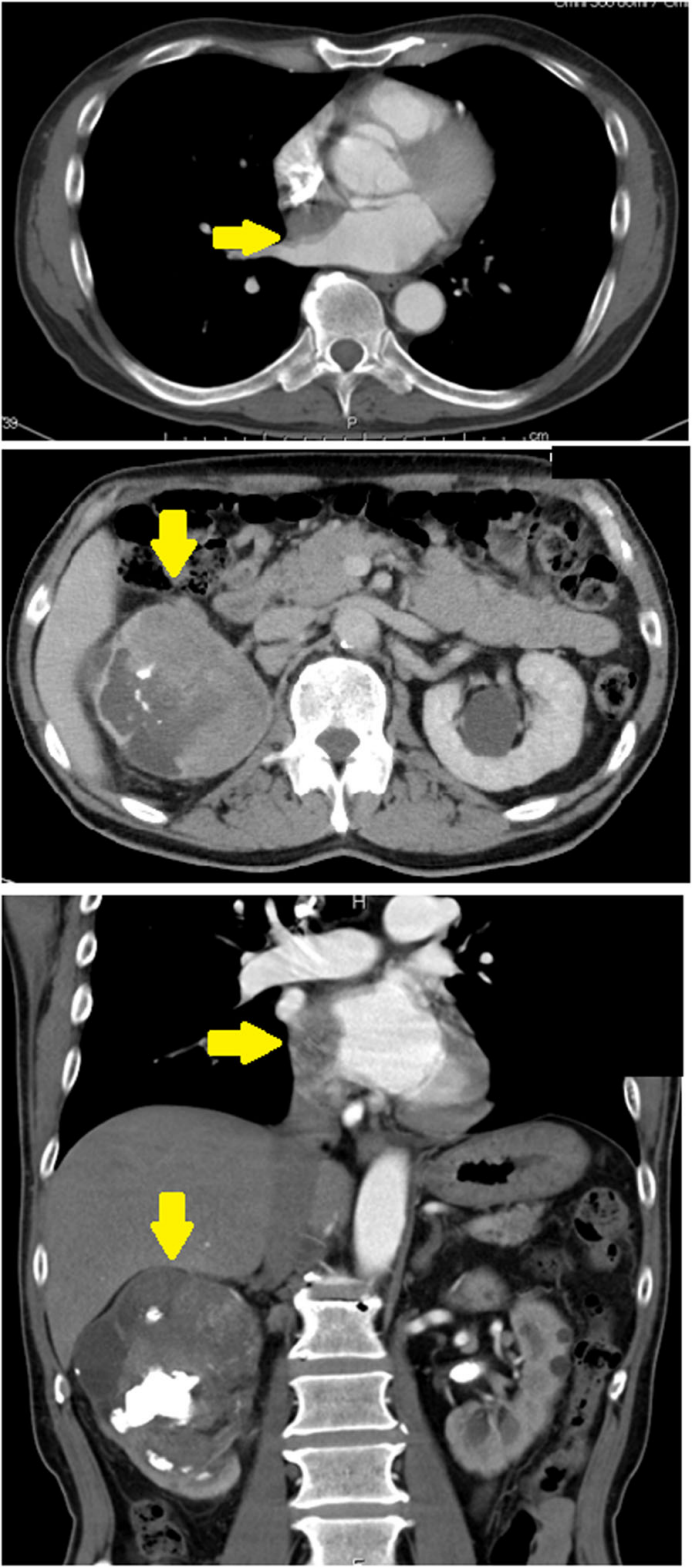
Computed tomography at the first examination. *Top*: computed tomography revealed a 32 mm hypodense mass (*yellow arrow* →) between the left and right atria. *Middle*: computed tomography showed an 86 mm hypodense mass (*yellow arrow*↓) located in the right kidney and extending into the right renal pelvis. *Bottom*: both cardiac (*yellow arrow*→) and renal masses (*yellow arrow*↓) are visible
